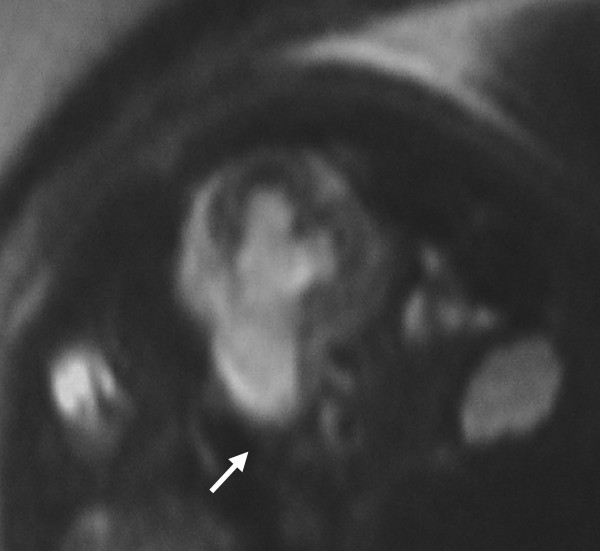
Fetal Magnetic Resonance Imaging (MRI) imaging: T2-weighted sequences. Axial Half Fourier Rapid Acquisition with Relaxation Enhancement (RARE) T2-weighted images shows dilation of the middle third of the ureter and coexist homolateral upstream hydronephrosis.
